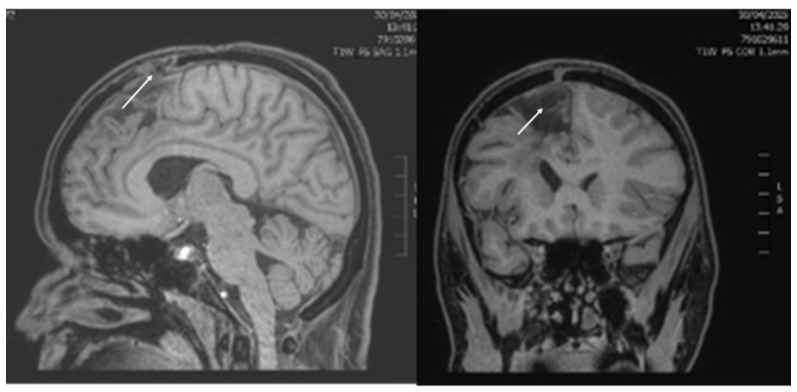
Brain MRI (T1-weighted images) upon the initial admission to the ICU due to status epilepticus. Right parietal lobe and corpus callosum postsurgical porencephaly with peripheral gliosis, and a small heterogenous high signal mass, indicating possible tumor recurrence (marked with white arrow).
